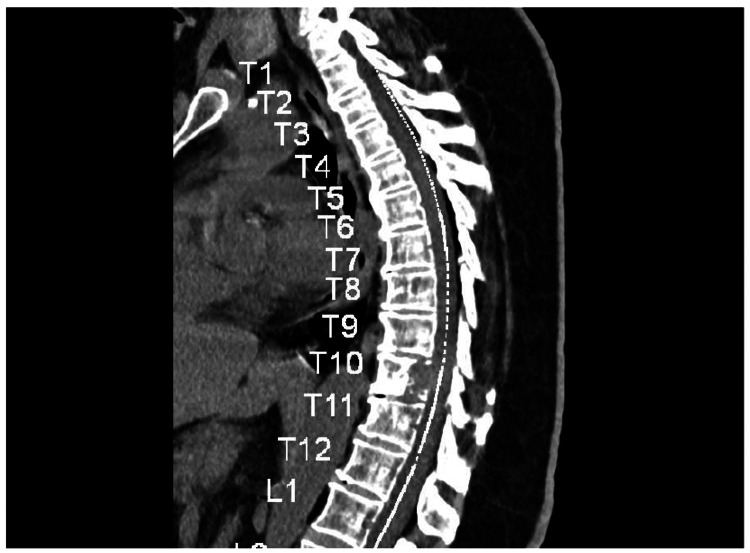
Sagittal CT spine showing a lytic lesion at T10.
